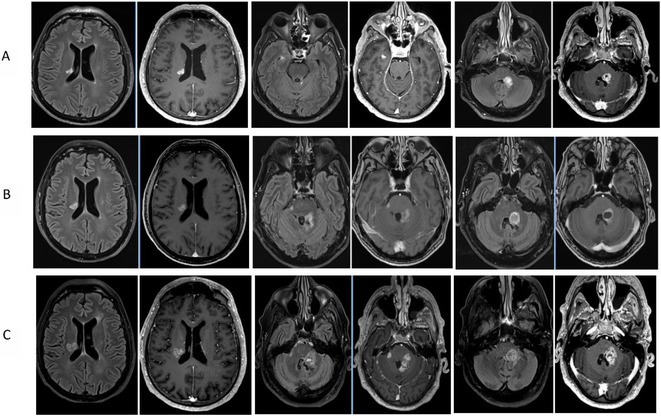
FIGURE 1 Progression within the months of the WML located in right temporal periventricular region and cerebellar peduncles. Contrast‐enhanced T1 and T2‐FLAIR acquired in (A) September 2023, (B) November 2023 and (C) January 2024.
